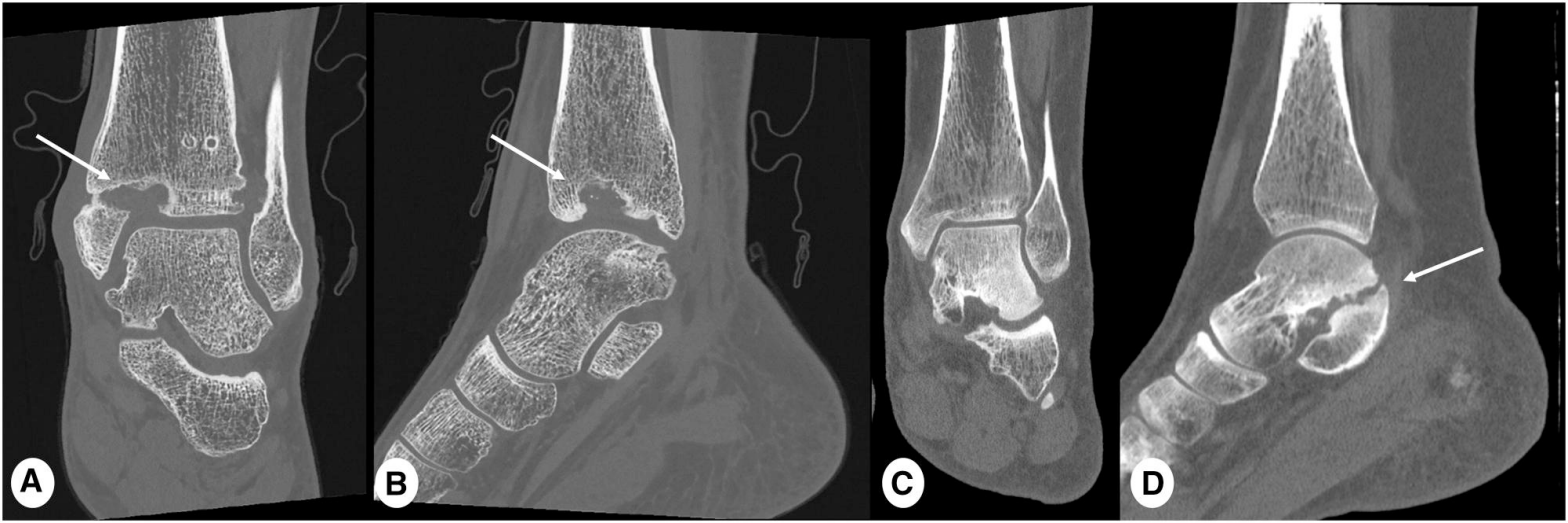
Example of assessment of osteomyelitis. (A) Coronal and (B) sagittal multiplanar reformat PCD-CT images (slice thickness 0.6 mm, reconstruction kernel Br76 and IR level 3, Q3) in 18 years old male with large tibial bone defect (arrows) in the distal left tibia extending to the medial malleolus caused by osteomyelitis. (C) Coronal and (D) sagittal EID-CT images reformats (slice thickness 0.75 mm, reconstruction kernel Ur77 and IR 3) in 18 years old male after surgical correction of talocalcaneal coalition (thick arrow). Note increase spatial resolution and definition of bone contours and spongiosa in PCD-CT image compared to EID-CT image. Moreover total radiation exposure was lower in PCD-CT than EID-CT with total dose-length product (DLP) was 75 mGy×cm and 327 mGy×cm for PCD-CT and EID-CT, respectively.
